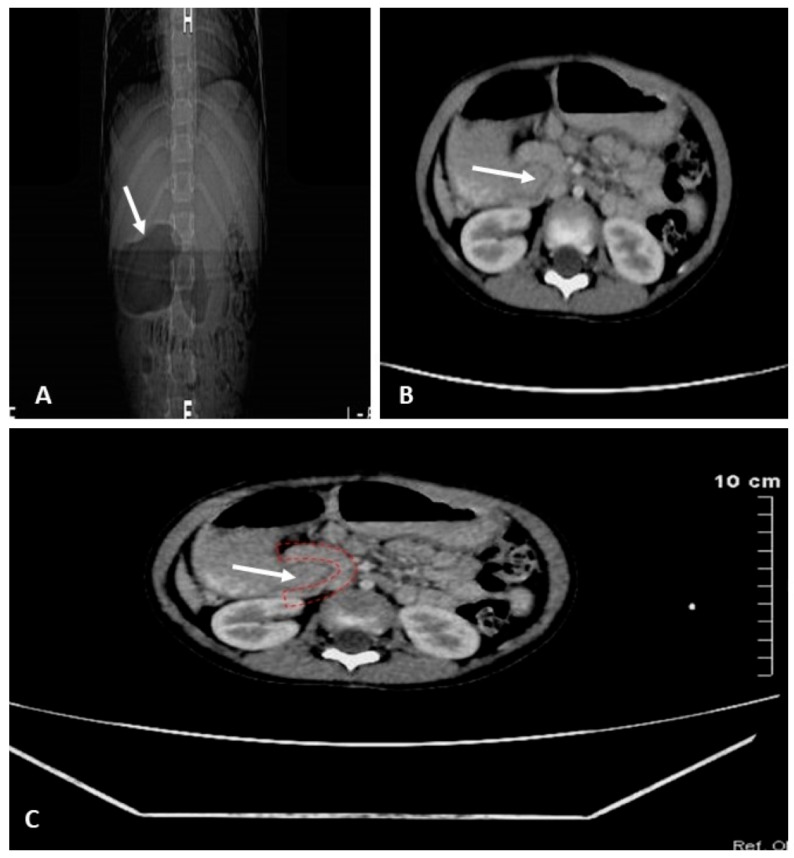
Abdominal CT: Cross-sectional imaging demonstrated pancreatic tissue partially encircling the second portion of the duodenum (arrows), associated with marked dilation of the stomach and proximal duodenum. (A): CT Scanogram: Dilatation of the duodenum and gastric antrum. (B,C): Annular pancreas (red) causing extrinsic compression of the duodenum with resulting proximal (prestenotic) dilatation (arrows).
